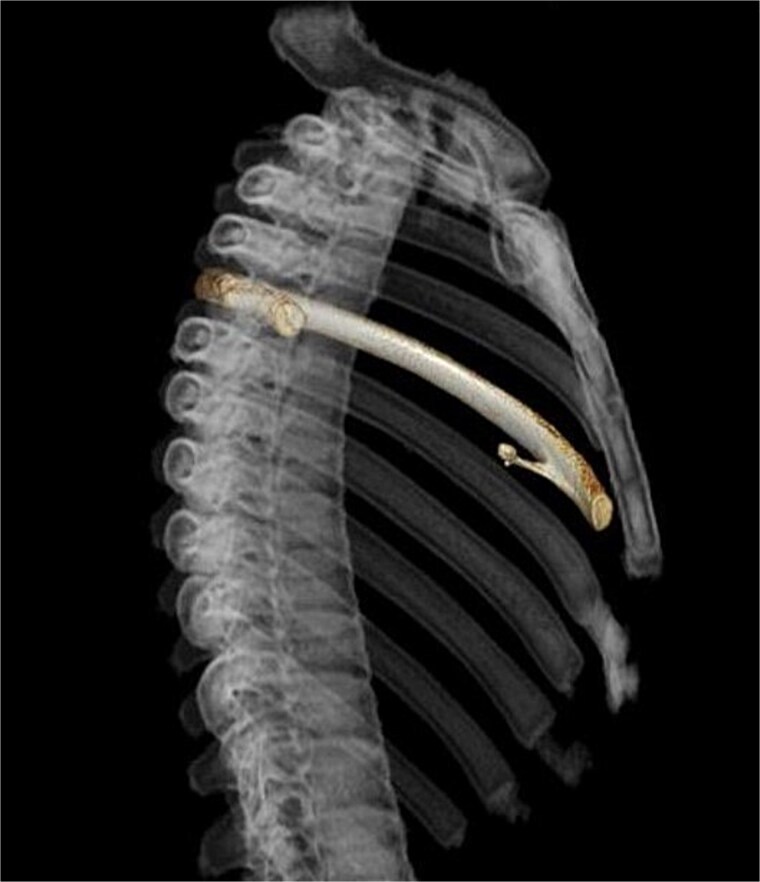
Three-dimensional computed tomography. Slim and pedunculated osteochondroma arising from the anterior fifth rib into the thoracic cavity.
